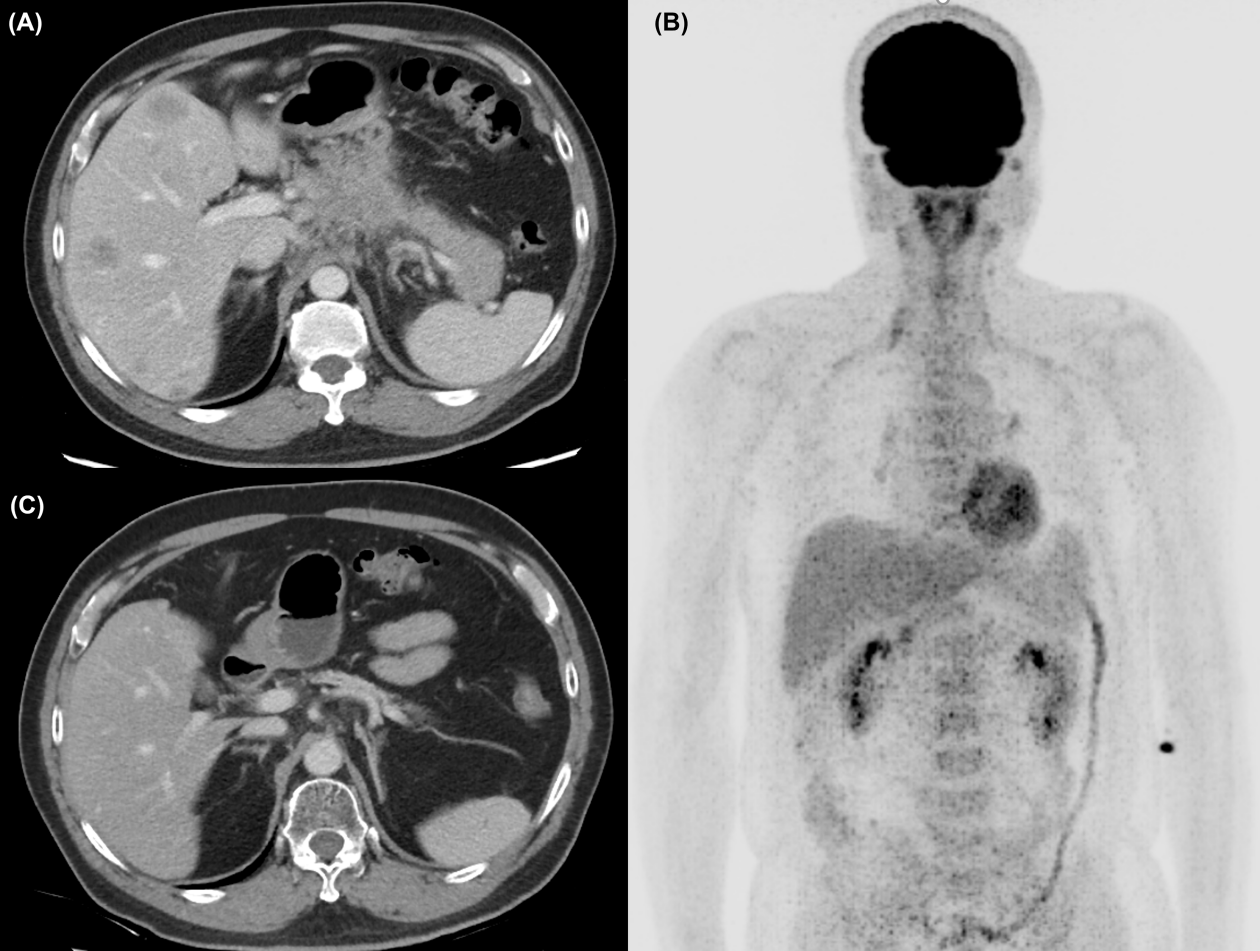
(A) The abdominal computed tomography (CT) scan revealed a 5-cm mass at the pancreatic body with liver metastases at diagnosis. (B) After chemotherapy and RFA of liver metastasis, no [18F]-FDG uptake was identified at PET scan. (C) At 32 months after initial diagnosis, the patient remains disease-free under maintenance nivolumab alone.
